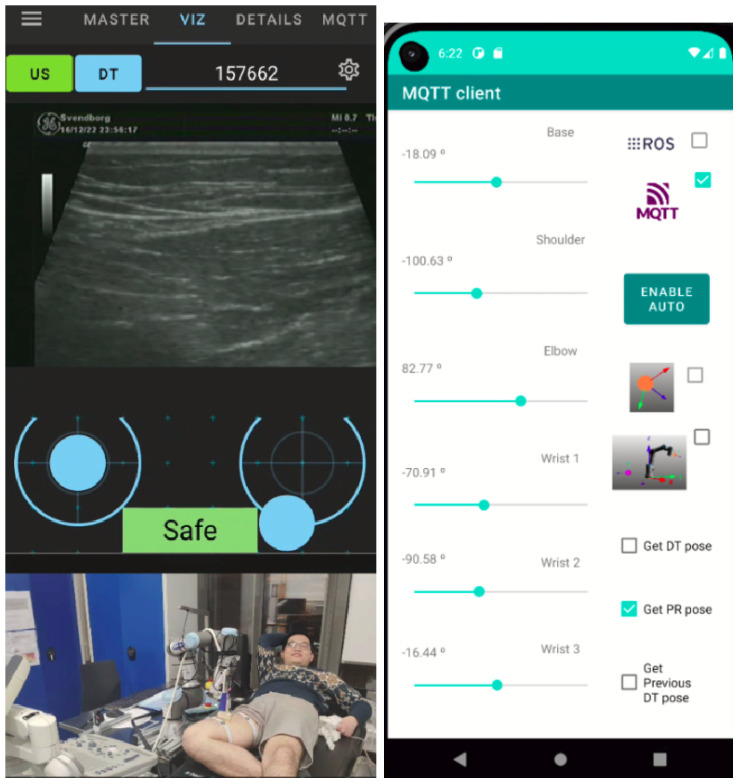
RT for robotic ultrasound scan on the subject’s leg using the ad hoc ROS-Mobile. The integrated MQTT client synchronizes the movements of both DT and RA.
